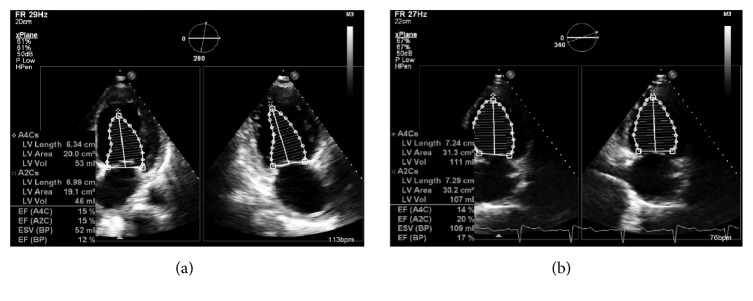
Echocardiography demonstrating decreased ejection fraction on admission (a) and two months later (b).
